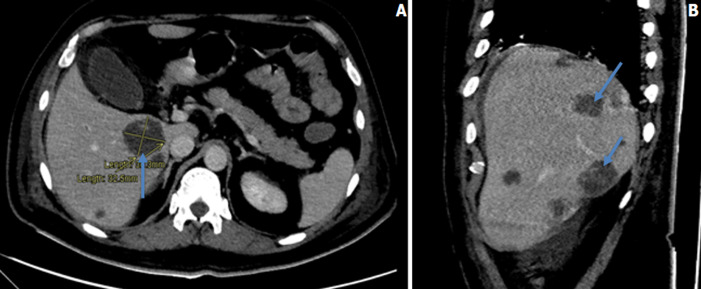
A, B) CT-abdomen: multiple non-enhancing cystic liver lesions with largest measuring 3.6 x 3.2 cm
